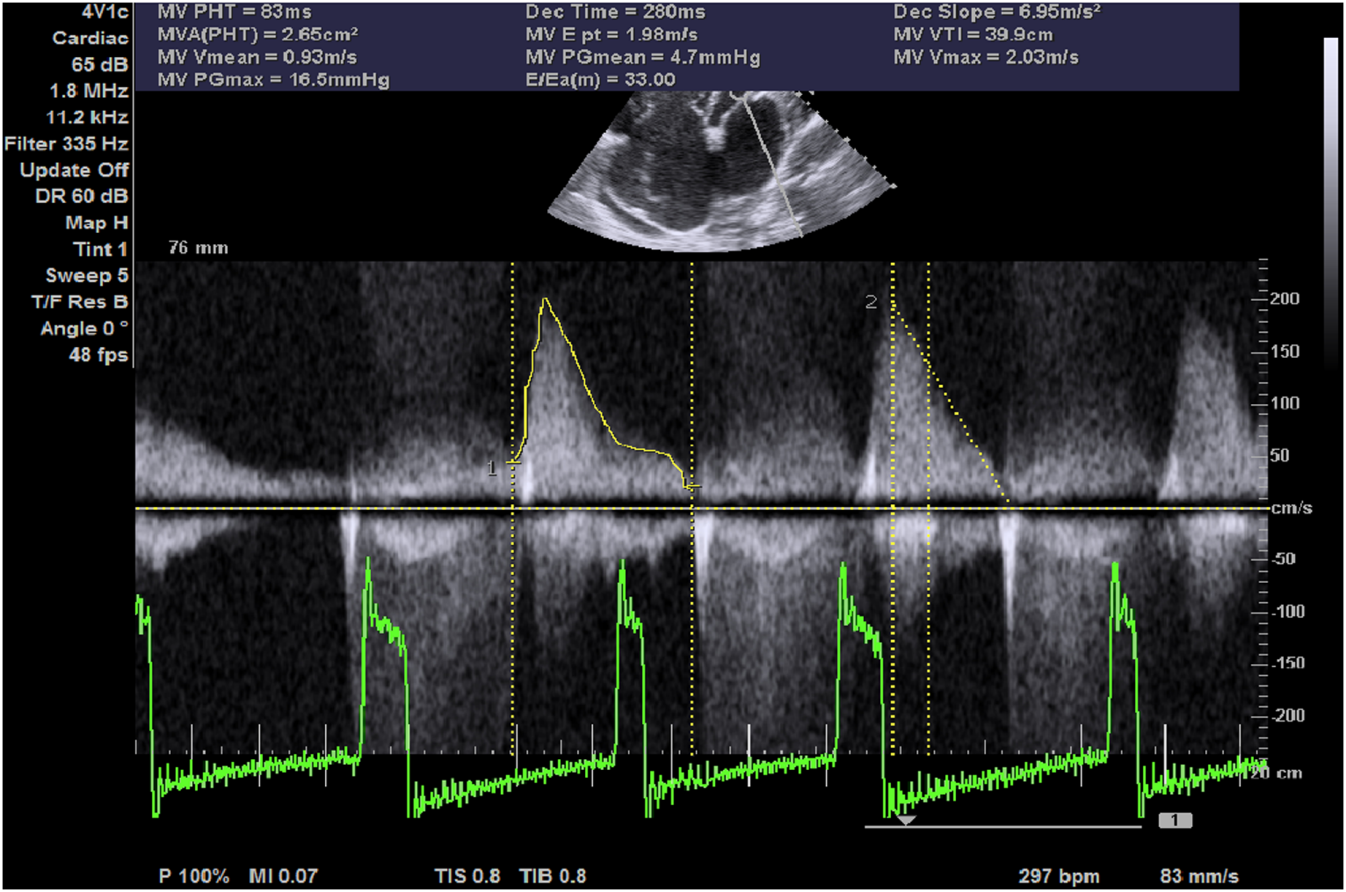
Epical 4 chamber echocardiogram showing continuous wave Doppler across the mitral valve with pressure halftime measurement and estimated mitral valve area. MAV PHT, mitral valve area derived from pressure half time; MV VTI, mitral valve velocity time integral; MVPGmax, mitral valve peak gradient; MVPHT, mitral valve pressure halftime.
